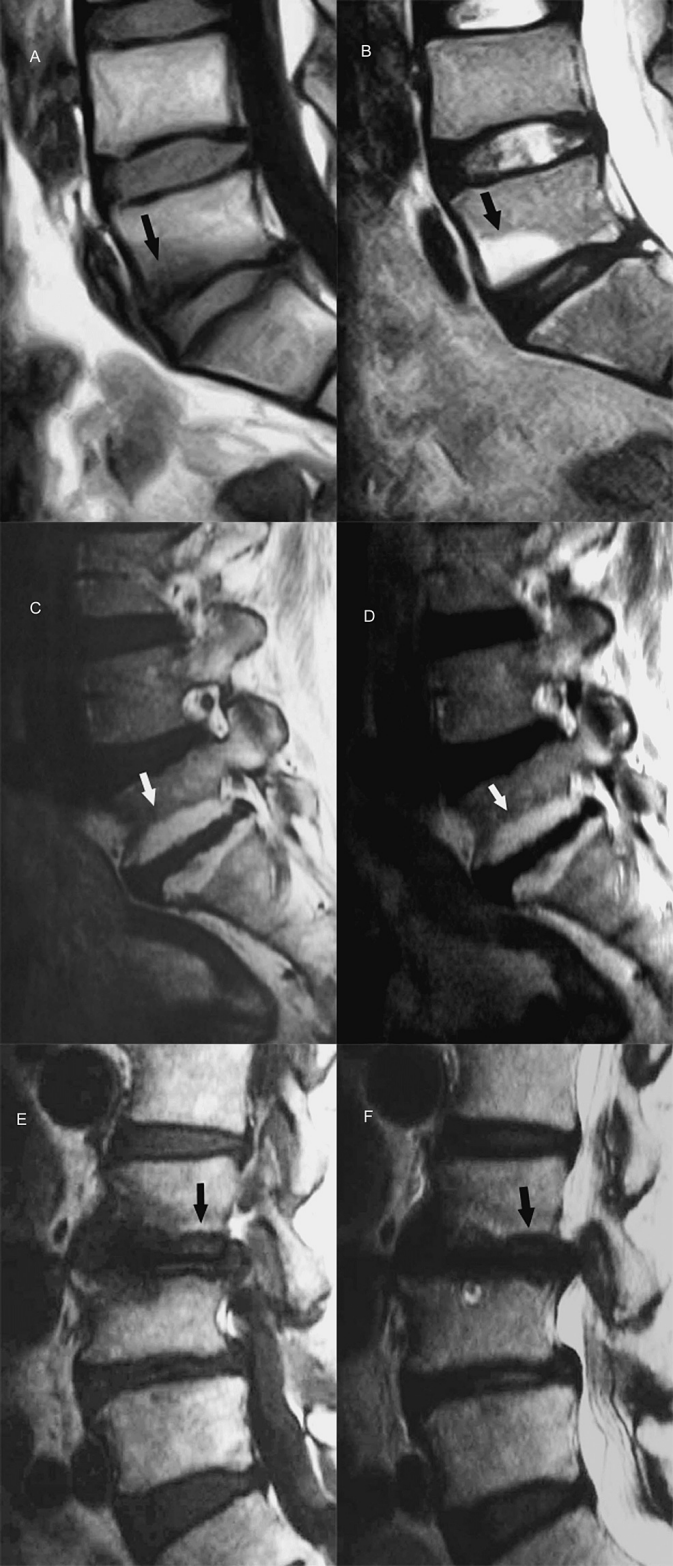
End-plate changes. (A, B) Sagittal T1- and T2-weighted MR images show Modic type 1 end-plate marrow changes (arrow) of low and high signal intensity, respectively corresponding to the formation of fibrovascular tissue. (C, D) Sagittal T1- and T2-weighted MR images show Modic type 2 end-plate marrow changes (arrow) of high signal intensity corresponding to fatty marrow. (E, F) Sagittal T1- and T2-weighted MR images show Modic type 3 end-plate marrow changes (arrow) of low signal intensity corresponding to the formation of fibro-osseous tissue.
